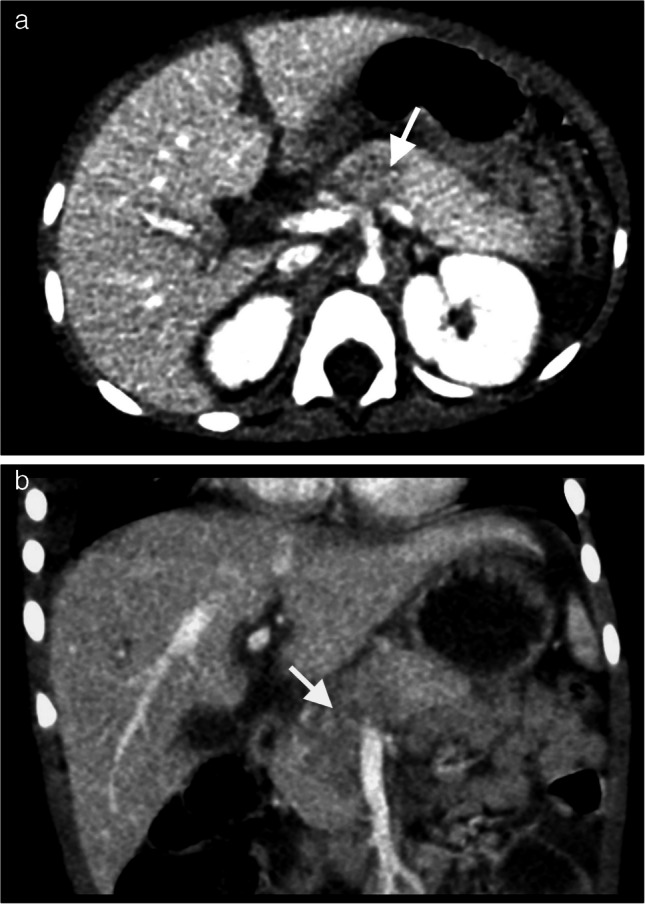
A 3-year-old female with multiple abdominal injuries from physical abuse including grade II left hepatic lobe lacerations, right adrenal hematoma, and a pancreatic contusion with elevated serum lipase. CT of the abdomen and pelvis with IV contrast was obtained in the portal venous phase. a Axial image at the level of the pancreas shows focal pancreatic body contusion (*white arrow*). b Coronal image shows the pancreatic body contusion (*white arrow*)
